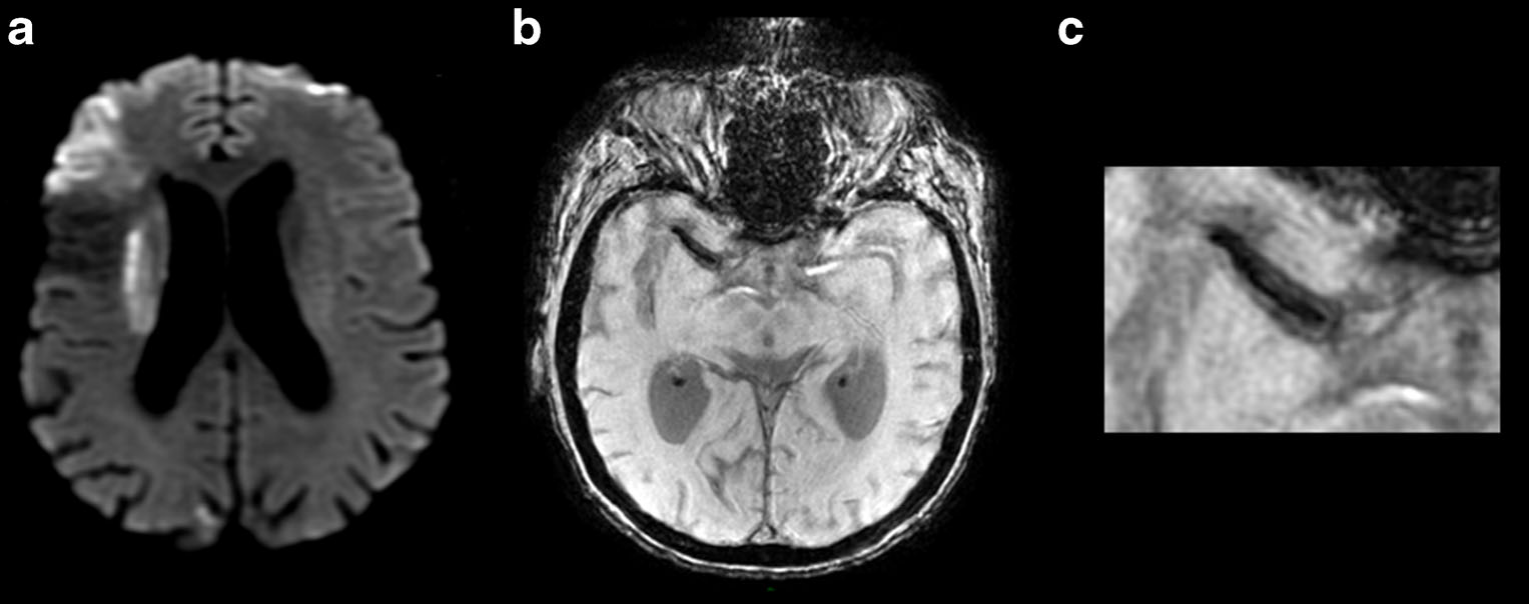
An 85-year-old patient presenting left hemibody deficit on DWI (a) and SWI (b and c) sequences in the axial plane. a Acute superficial sylvian and deep right ischaemic event. b and c Long thrombus in the M1 segment of the right middle cerebral artery with a two-layered susceptibility sign
